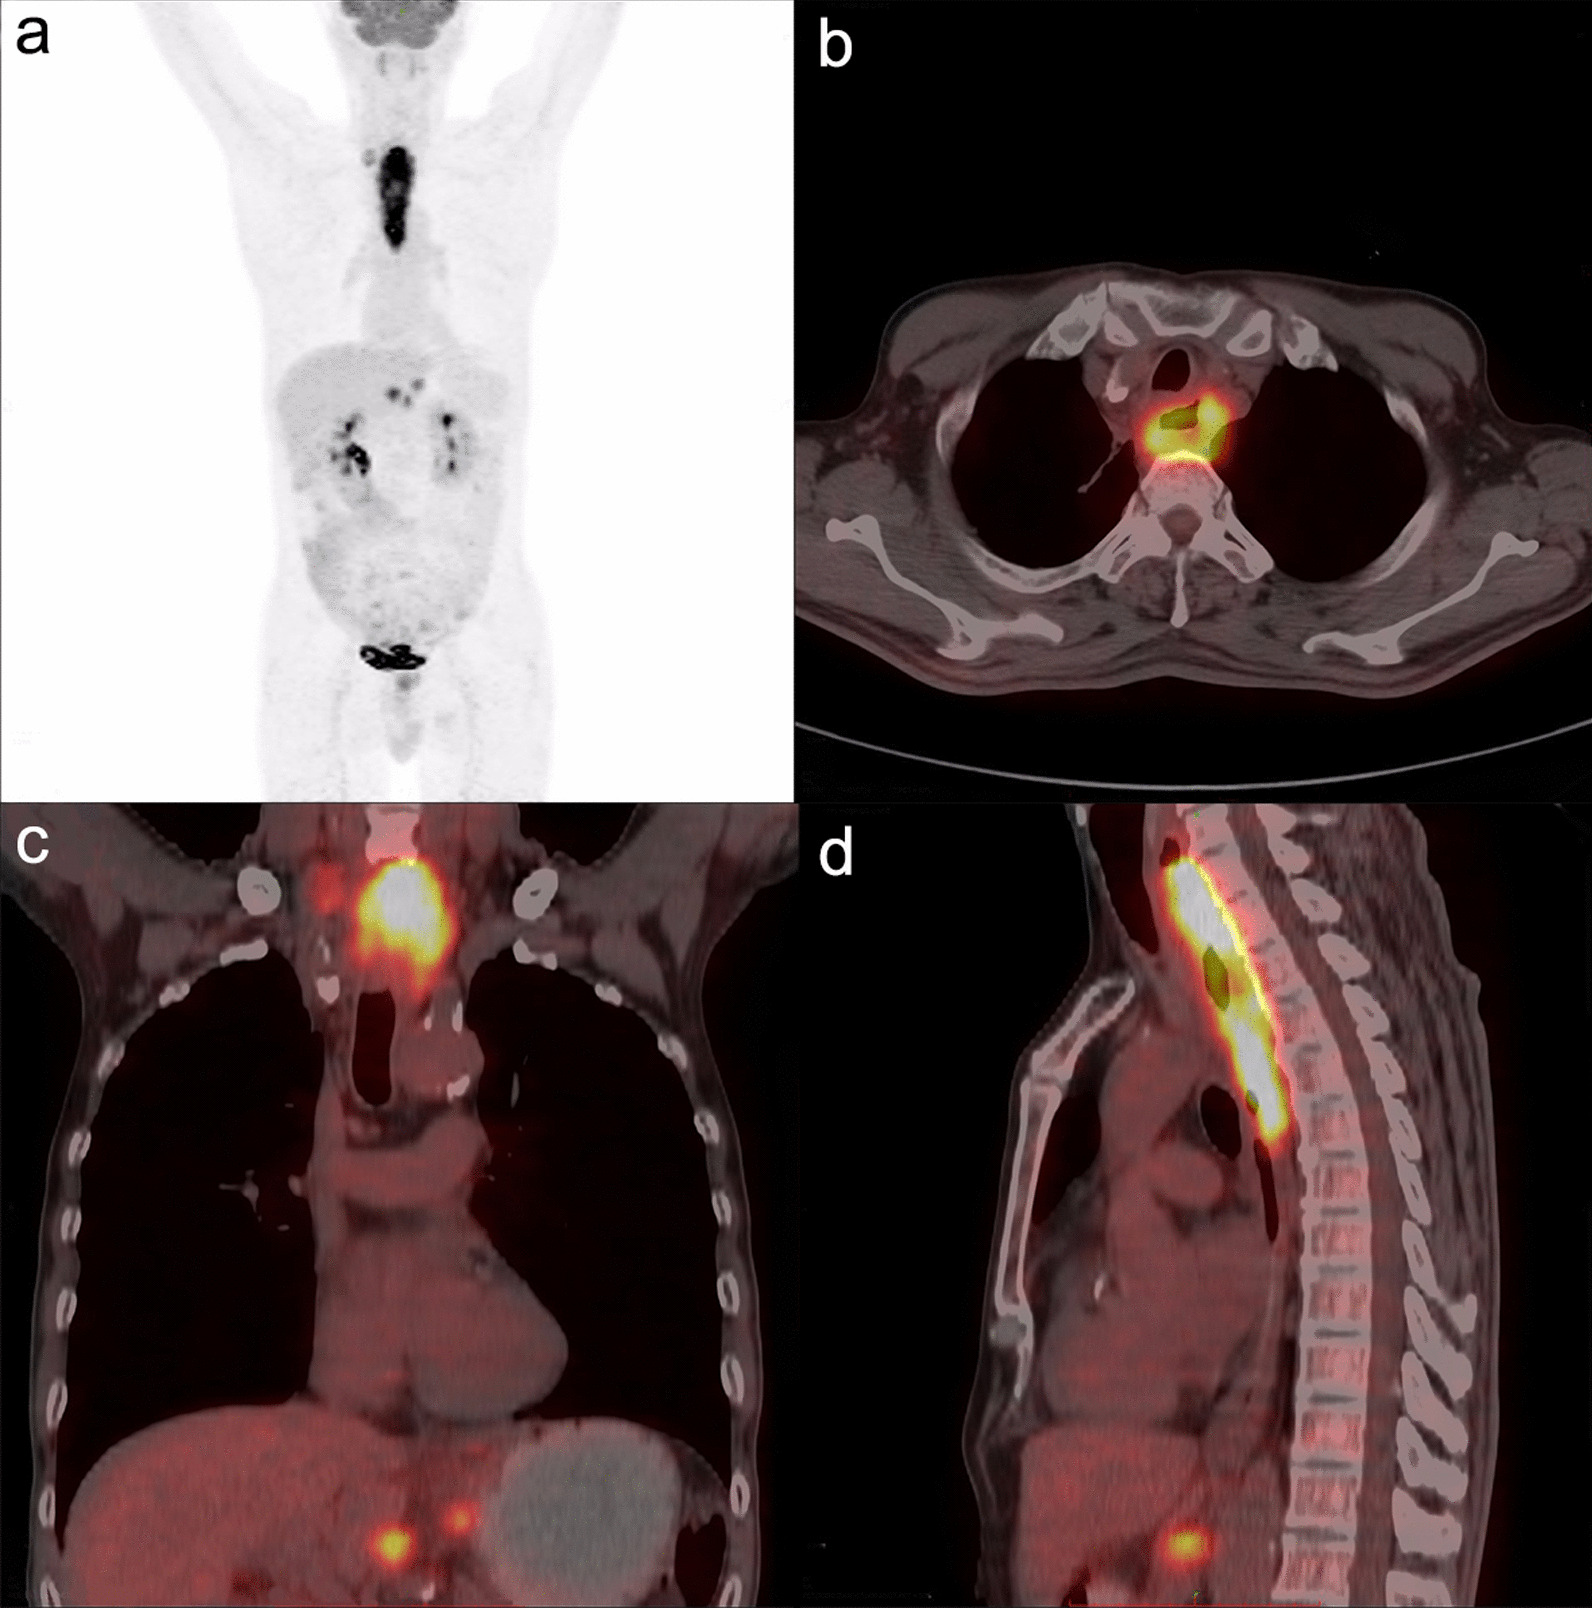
A 60-year-old male with esophageal squamous cell carcinoma. The ^18^F-FDG PET/CT fusion image showed thickening of the middle and upper esophageal wall characterized by increased metabolism. SUV_Tumor_, MTV and TLG were 17.2, 66.41 cm^3^ and 579.6 g, respectively. Lymph node metastasis in the right cervical root and hepato-gastric space, and metabolism increased. The PLNS number was 4, and the SUV_LN_ and SD_max(LN-T)_ were 8.97 and 1.03 m^−1^, respectively. The patient received definitive chemoradiotherapy and died with an OS of 4 months
